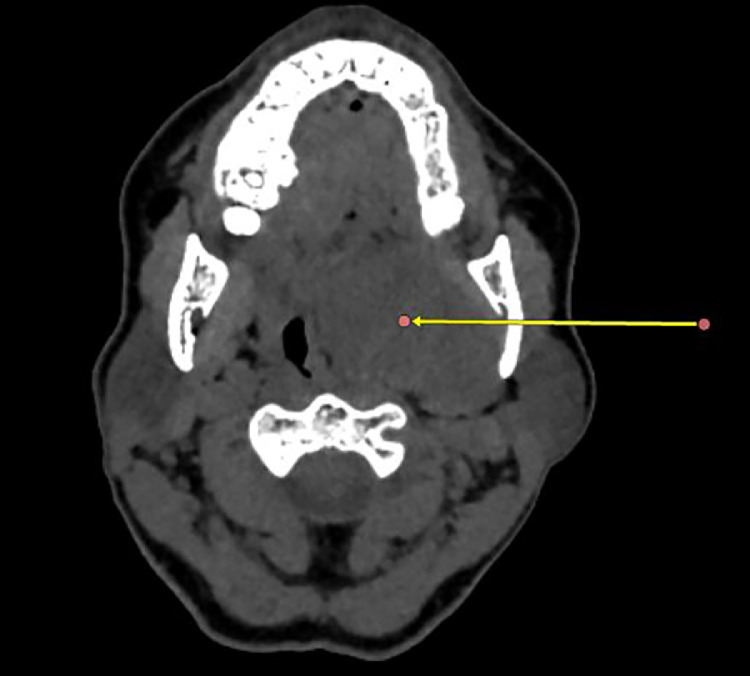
Axial CT scan, wide-window view, showing a well-bounded tissue mass in the left parapharyngeal space, separated from the deep lobe of the parotid gland by a fatty border .
